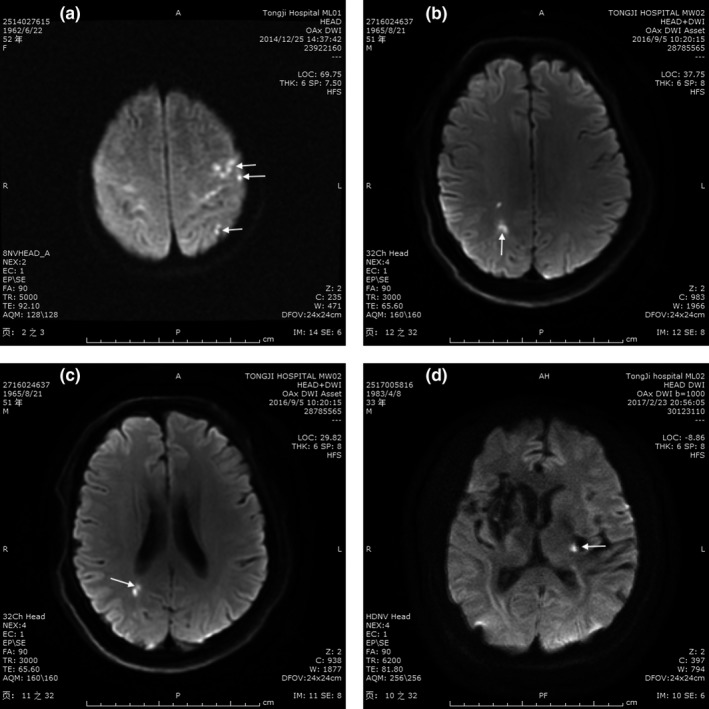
Representative cases showing different infarct patterns in diffusion‐weighted imaging (DWI). (a) Multiple small lesions located in cortex; (b) multiple small corti‐subcortical lesions; (c) multiple small subcortical lesions; (d) single small deep lesion (white arrows)
